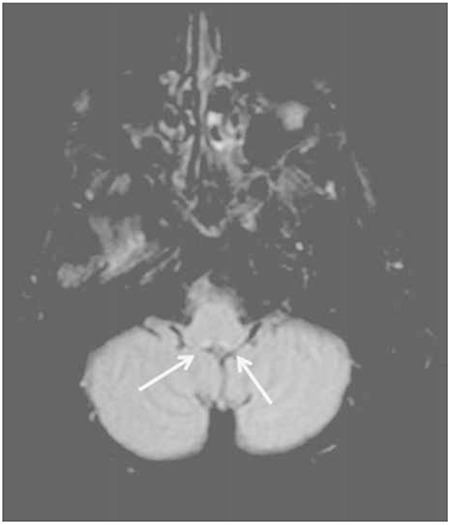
Control magnetic resonance imaging 2 weeks after the onset of the symptoms; fluid-attenuated inversion recovery image showing the diminution of increased signal around the aqueductus sylvii.
